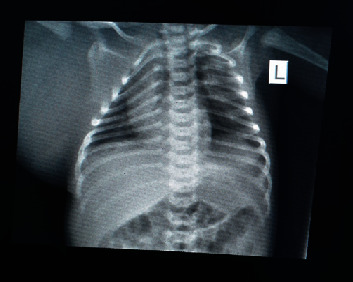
Plain chest X-ray showing homogenous soft tissue lesion left axillary region.
